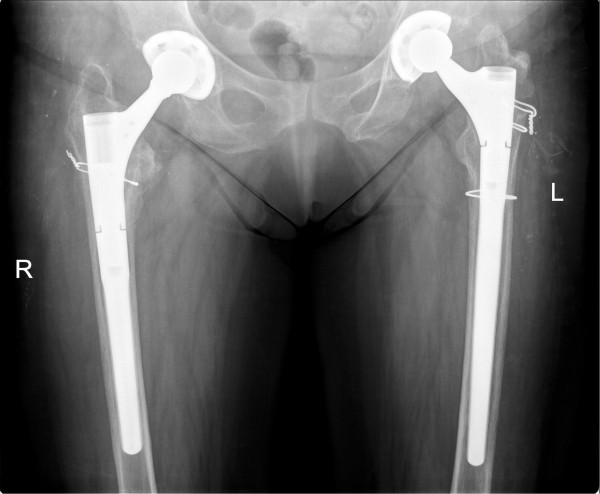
Postoperative X-ray at one year postoperative for her right hip (two years for her left hip) showing bilateral primary total hip arthroplasty with uncemented acetabular shell and modular femoral components with radiographic union for both pertrochanteric fractures.
